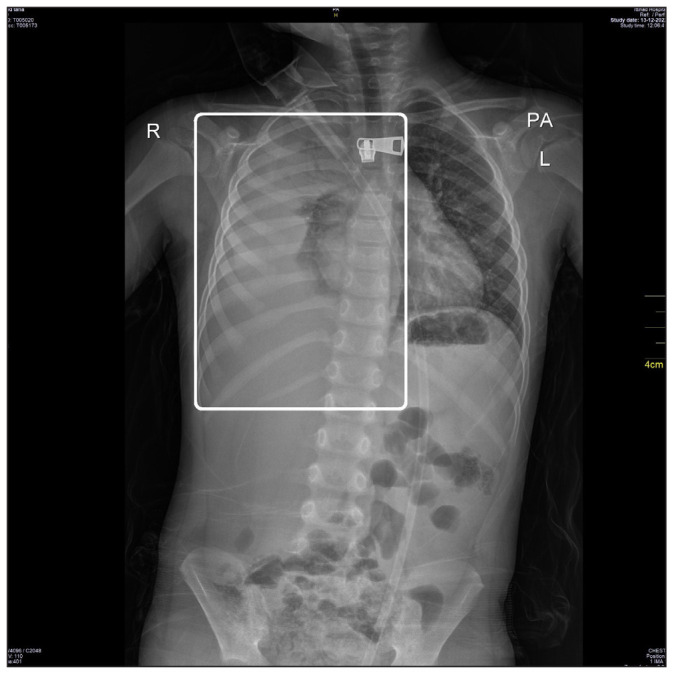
The patient’s second X-ray image demonstrating a right-sided pleural effusion (white rectangle).
